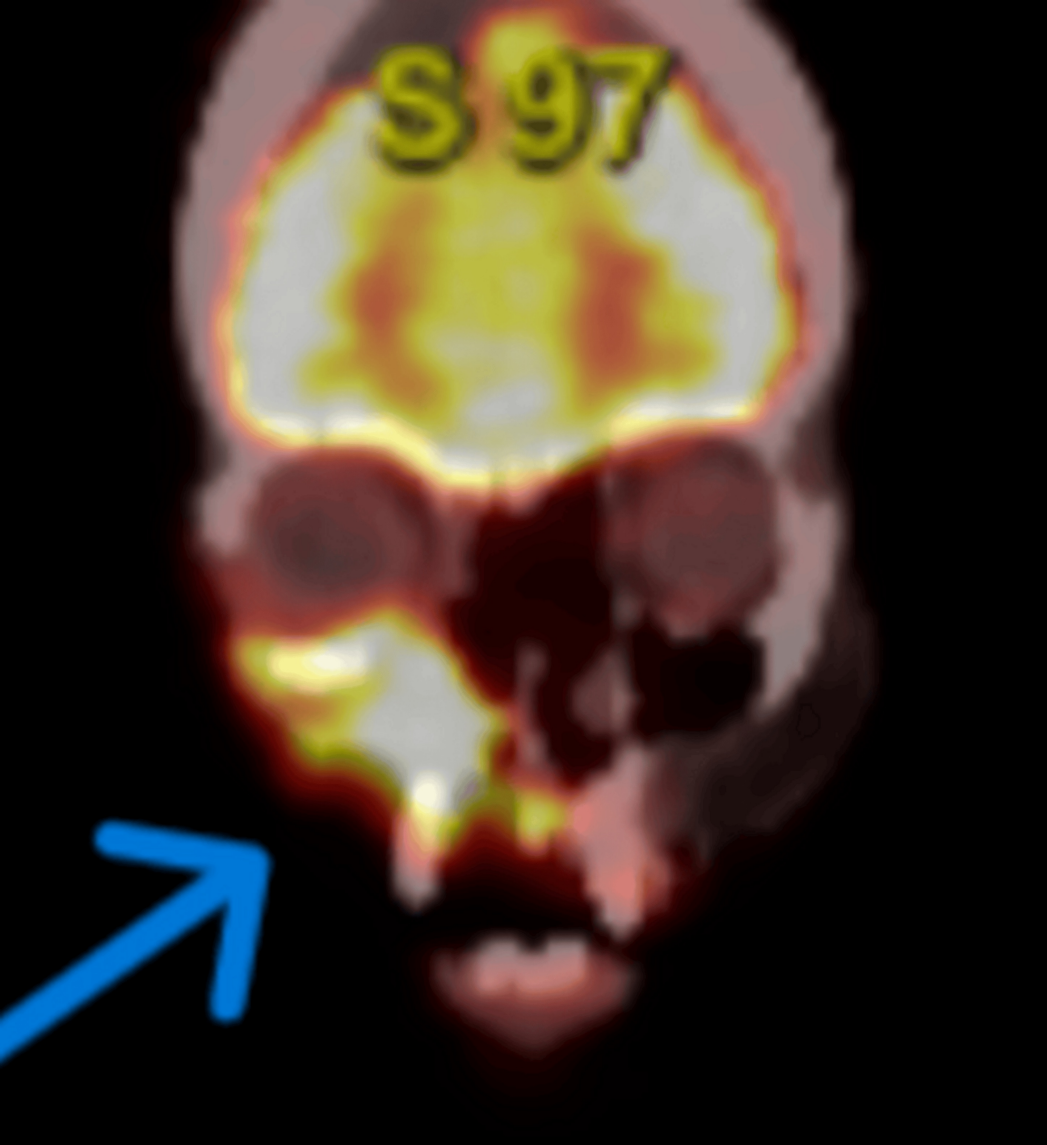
Coronal PET-CT image showing decreased metabolic activity of the facial tumor. Three-month follow-up PET-CT revealed a substantial reduction in fluorodeoxyglucose (FDG) uptake and volume of the right facial mass, consistent with metabolic and structural response to treatment.
